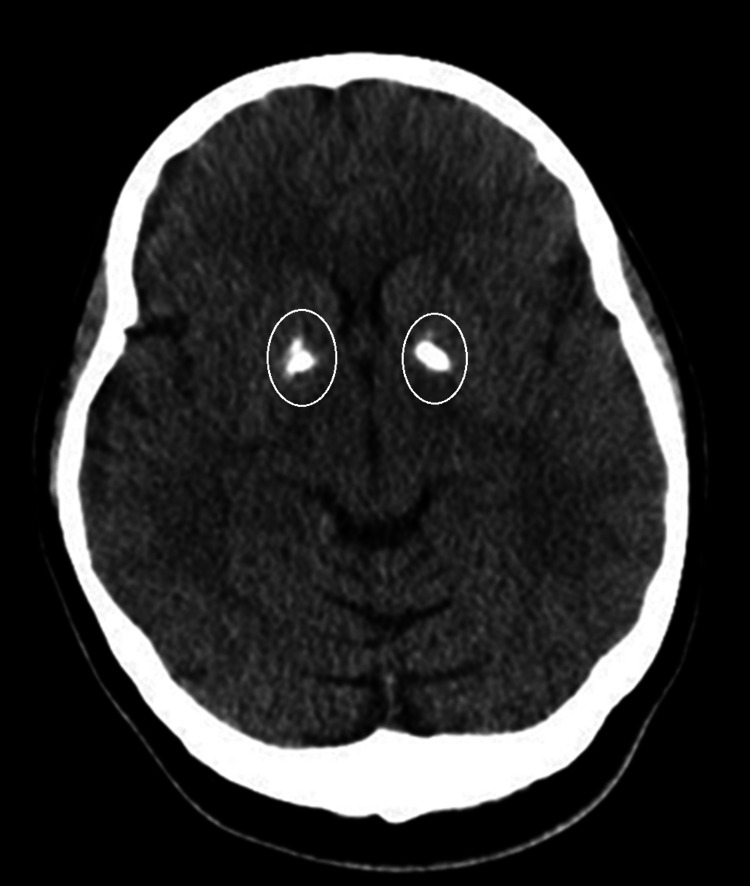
Axial non-contrast CT of the brain An axial CT image of the brain shows normal parenchymal structures. There is basal ganglia calcification (encircled), a common benign finding, with no associated mass effect or acute hemorrhage.
